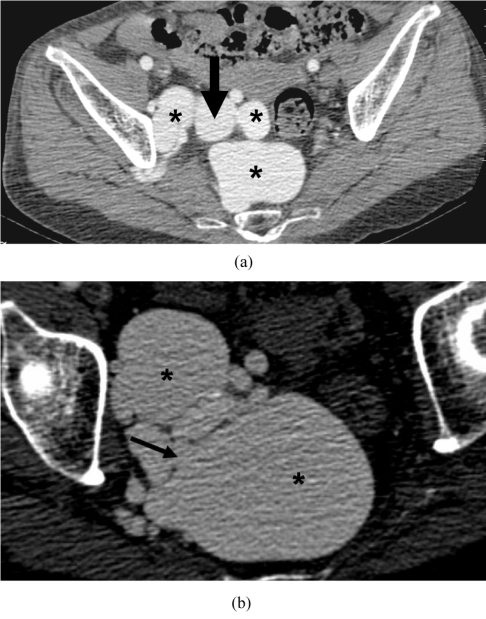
Contrast enhanced Axial CT at the level of the pelvis. (a) The enlarged tortuous right iliac artery (arrow) and right internal iliac veins (asterisk); (b) Axial image at a lower level shows the AV fistula (arrow) from aneurysmal right internal iliac artery into the internal iliac vein. Dilated veins on either side (asterisk).
